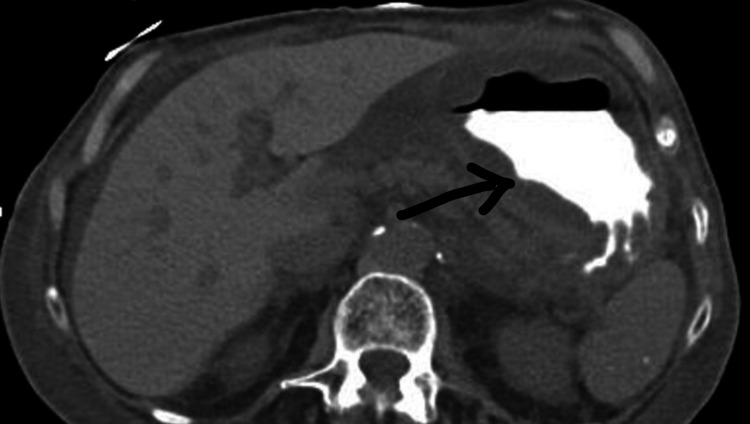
CT abdomen and pelvis on day three. Arrow showing gastric herniation and rotation into the patient’s parastomal hernia has been reduced. The stomach also looks decompressed. CT: computerized tomography.
